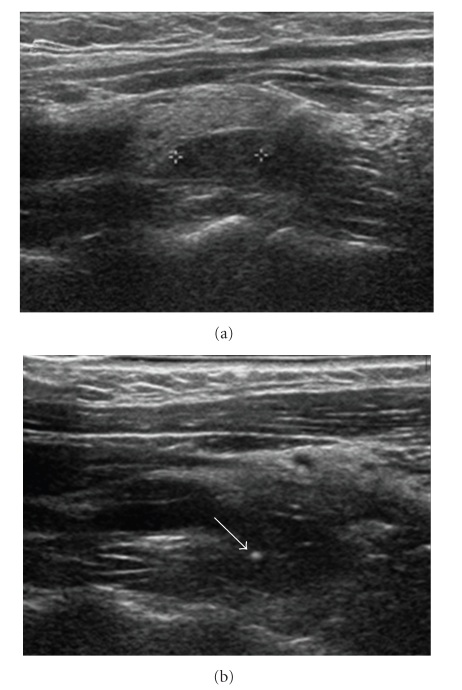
(a) Ultrasound of parathyroid adenoma. (b) Same patient, with guide wire in place (white dot).
